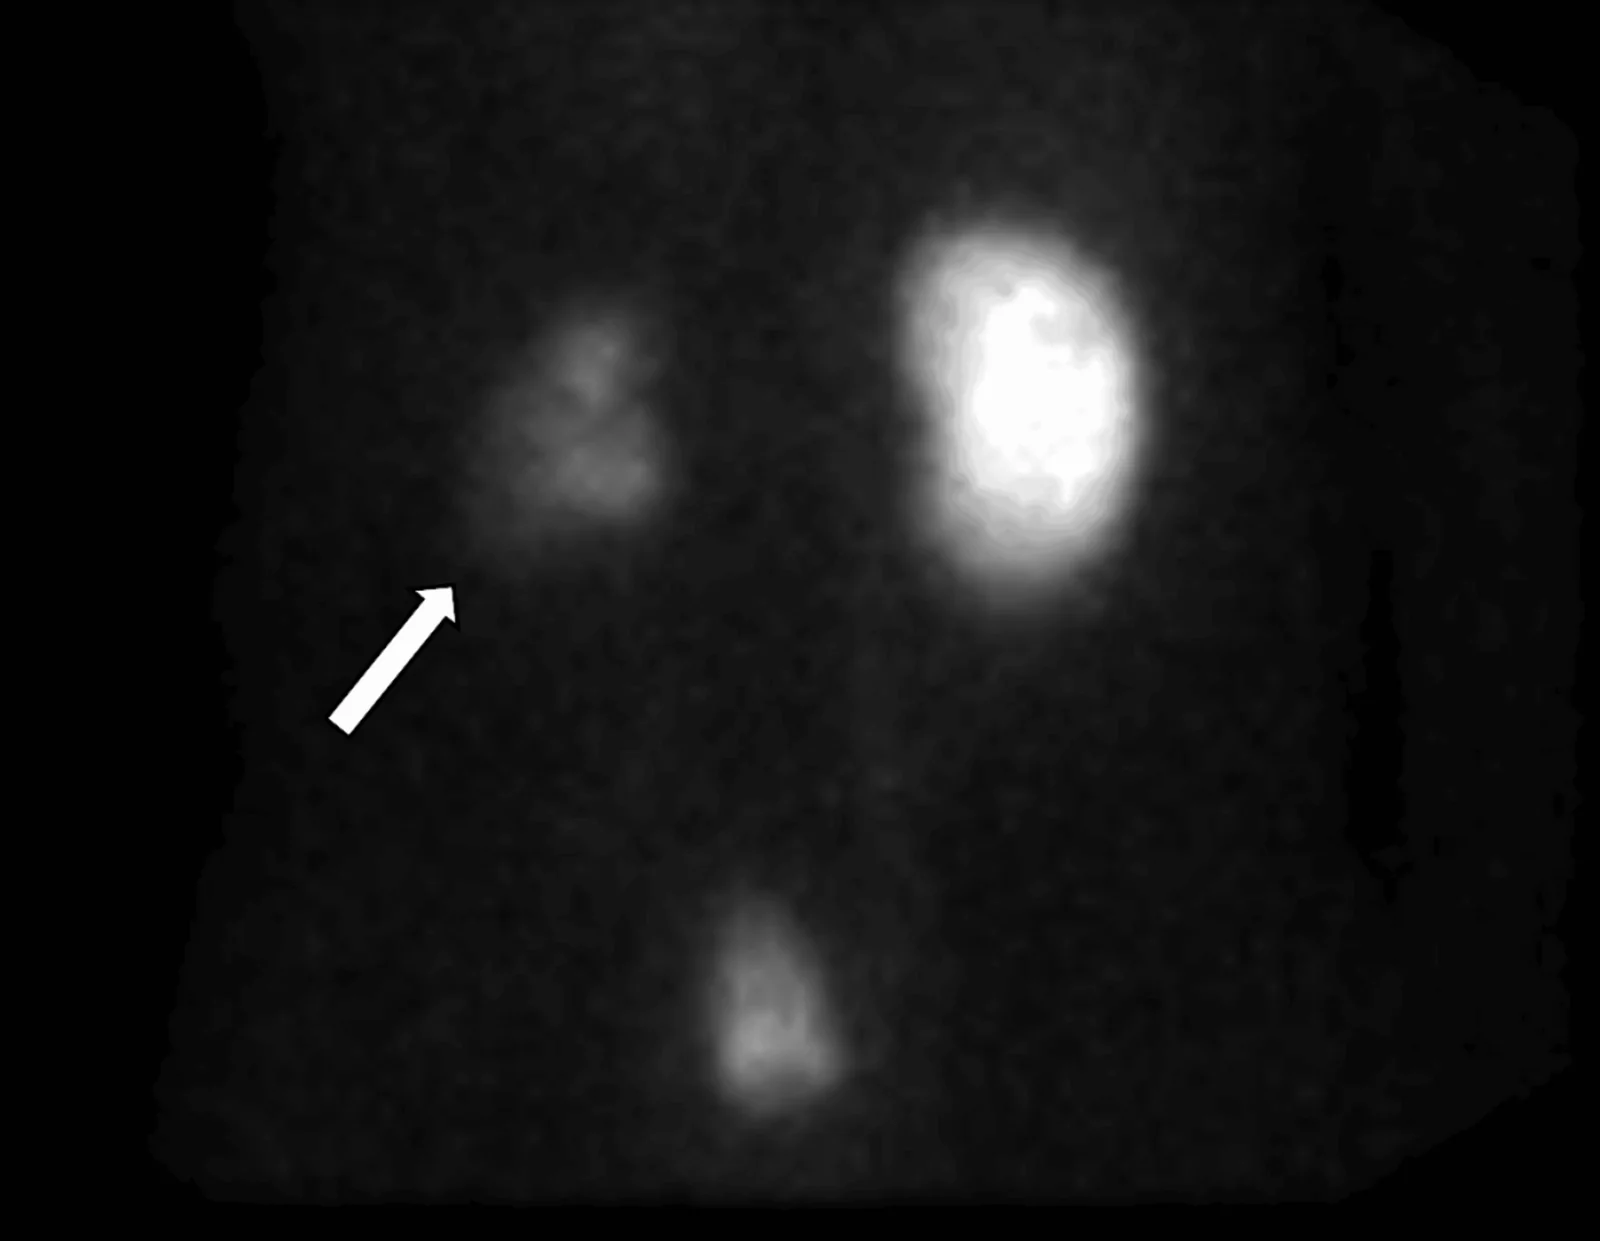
Technetium-99m MAG3 diuretic renogram demonstrating markedly asymmetric renal function. Posterior projection obtained during MAG3 renal scintigraphy shows markedly reduced radiotracer uptake in the left kidney (arrow), consistent with severely impaired perfusion and cortical function. Quantitative analysis demonstrated 14.5% differential function of the left kidney compared with 85.5% for the right kidney, supporting chronic irreversible obstructive nephropathy.
